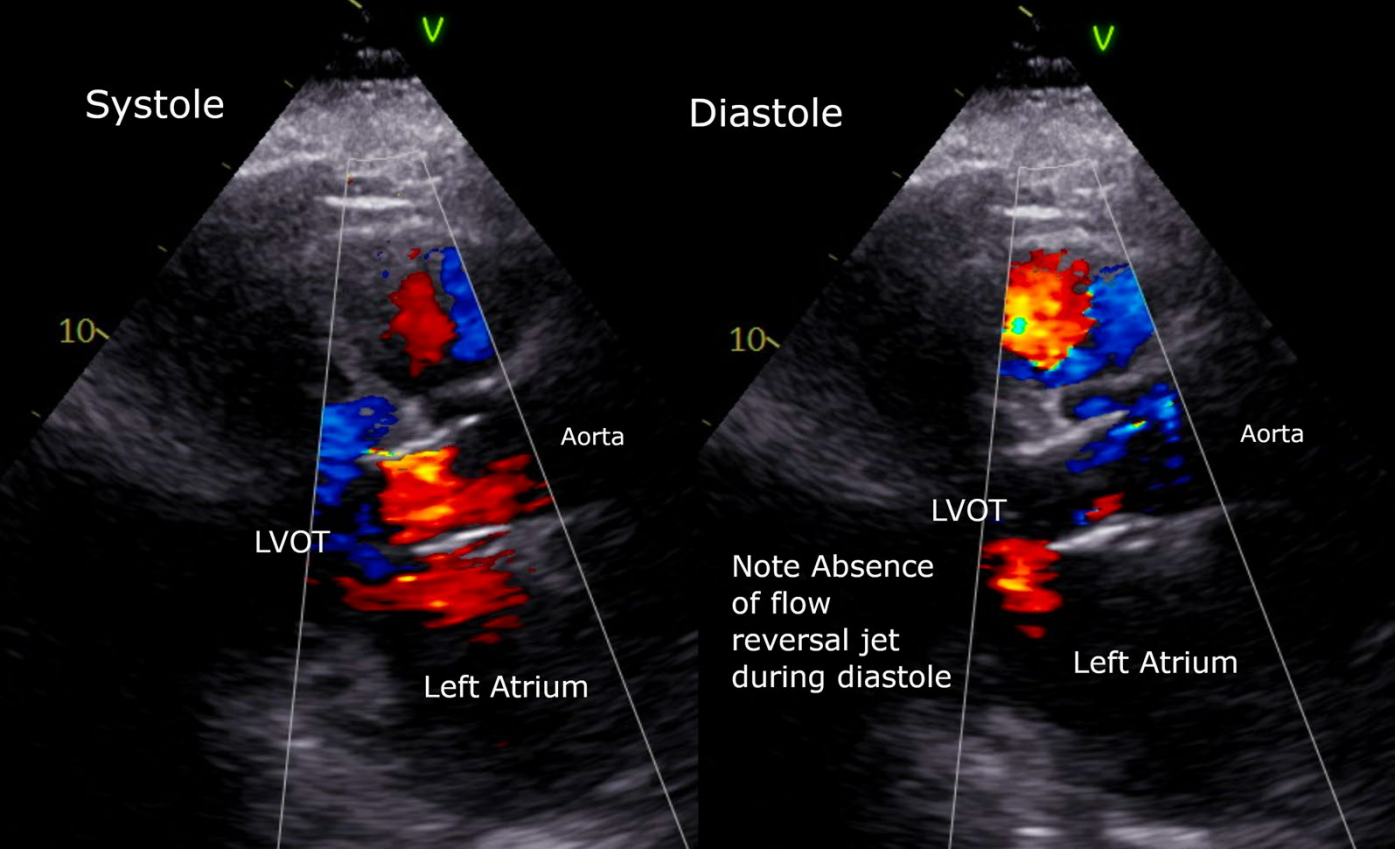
Post TAVR Transthoracic Echocardiogram showing good valve placement, and no residual paravalvular leak. Shown on the Left, Systolic flow through the valve and LVOT, and Right, no regurgitant jet, or paravalvular flow
